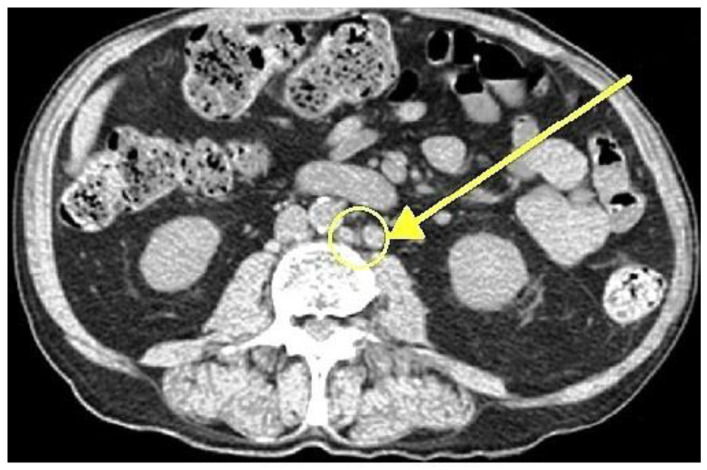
Axial contrast-enhanced CT scan demonstrating paraaortic lymphadenopathy (arrow indicating metastases of prostate cancer in lymph nodes).
